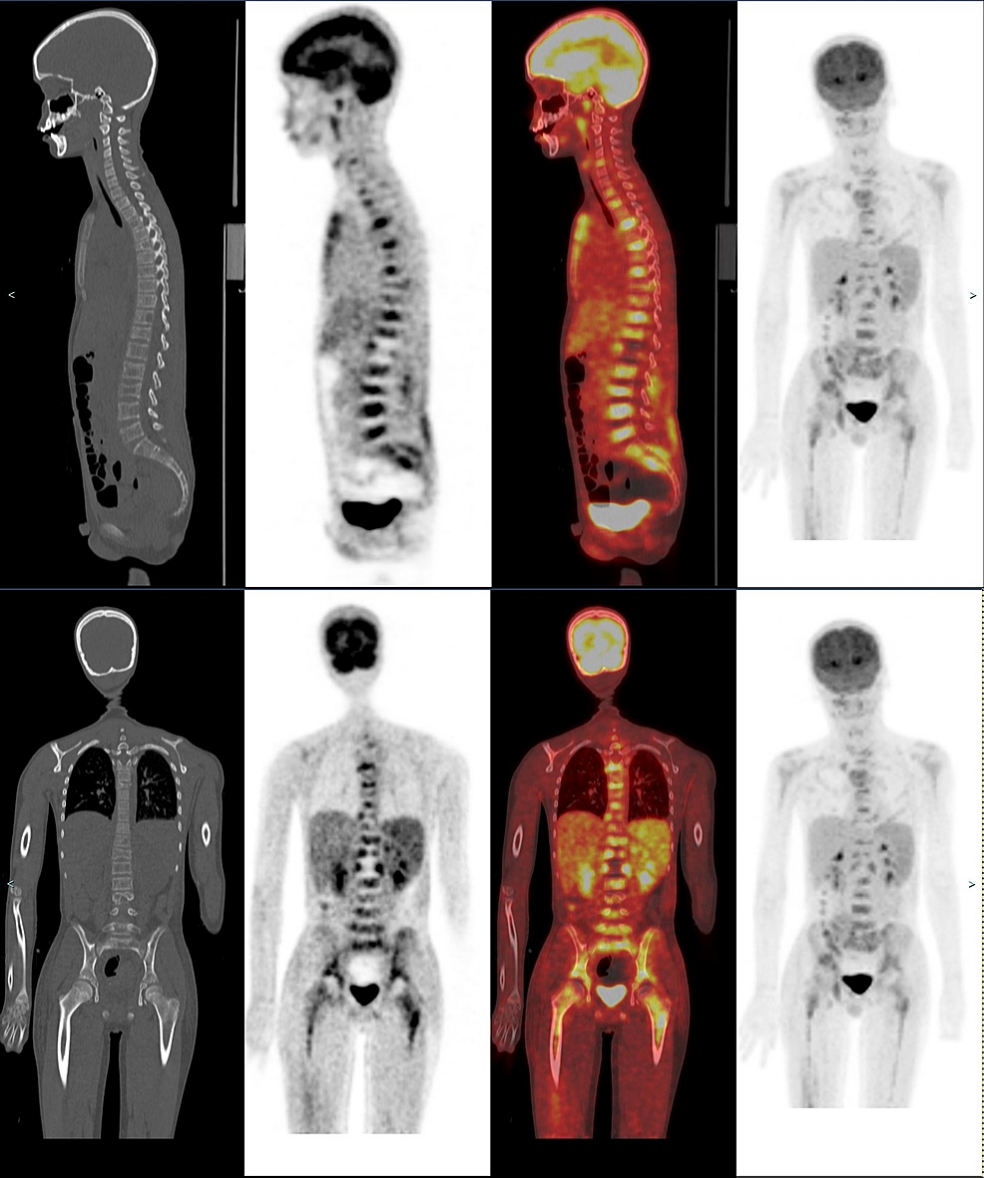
Skull to mid-thigh coronal and sagittal PET-CT (non-enhanced CT, attenuation correction PET slice and fusion images, from left to right) showed diffuse hypermetabolic marrow activity at multiple sites in the spine and pelvis, many of these corresponding to the lytic lesion. Note increased marrow metabolic activity in proximal humeri and femori bilaterally. Maximum intensity projection (MIP) image (last image, from left to right) showed no hypermetabolic focus in cervical, pulmonary regions, and solid abdominal viscera. Overall, these are most concerning for marrow metastatic disease (correlating with prior MRI) without primary site in neck, chest, abdomen, and pelvis.
